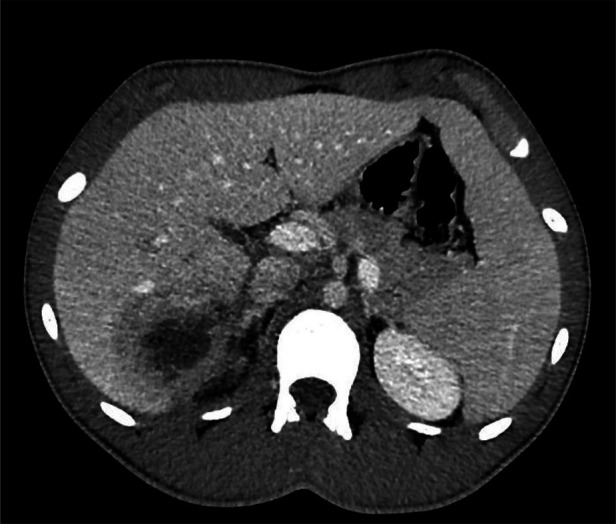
CT scan: Abscess (60 × 53 × 90 mm) in hepatic segments S6/S7, with necrotic components and intralesional air bubbles.
